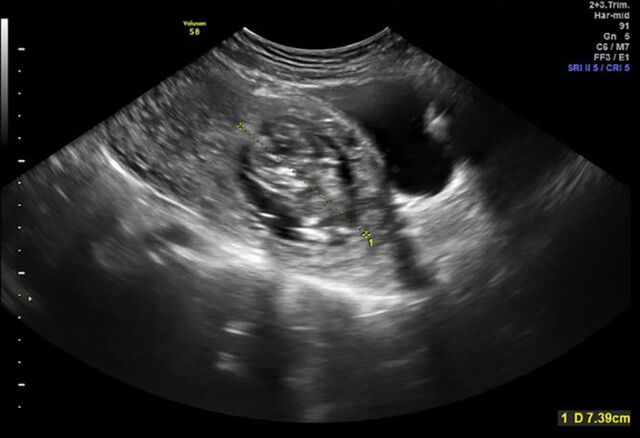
Ultrasound postamniocentesis at 14 weeks 1 day of gestation demonstrating interval decrease in the size of the cervical mass.
